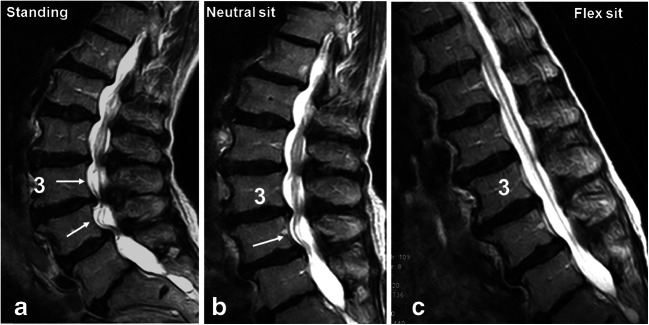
Sagittal T2-weighted upright MRI images of a single patient: a in standing position with evidence of serpentine-shaped RNRs (white arrows) cranially and caudally from the stenotic level L3/L4; b in neutral sitting position with RNRs (white arrow) only caudally from the stenotic level and c in flexed sitting position with no evidence of RNRs
